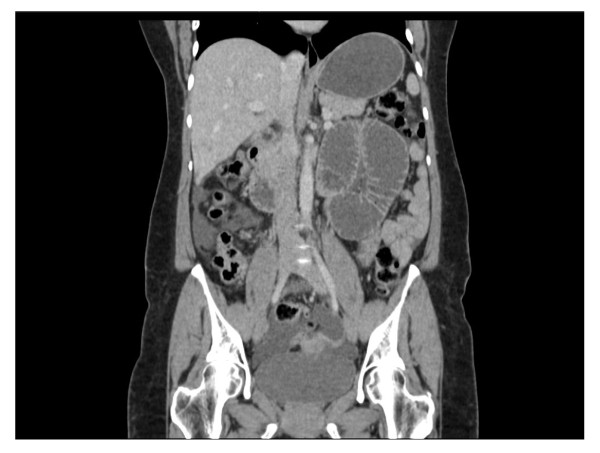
Computed tomography of the abdomen and pelvis, coronal section. A computed tomography scan taken on day 5, showing small bowel obstruction with proximal bowel dilatation and a transition point at the proximal jejunum.
